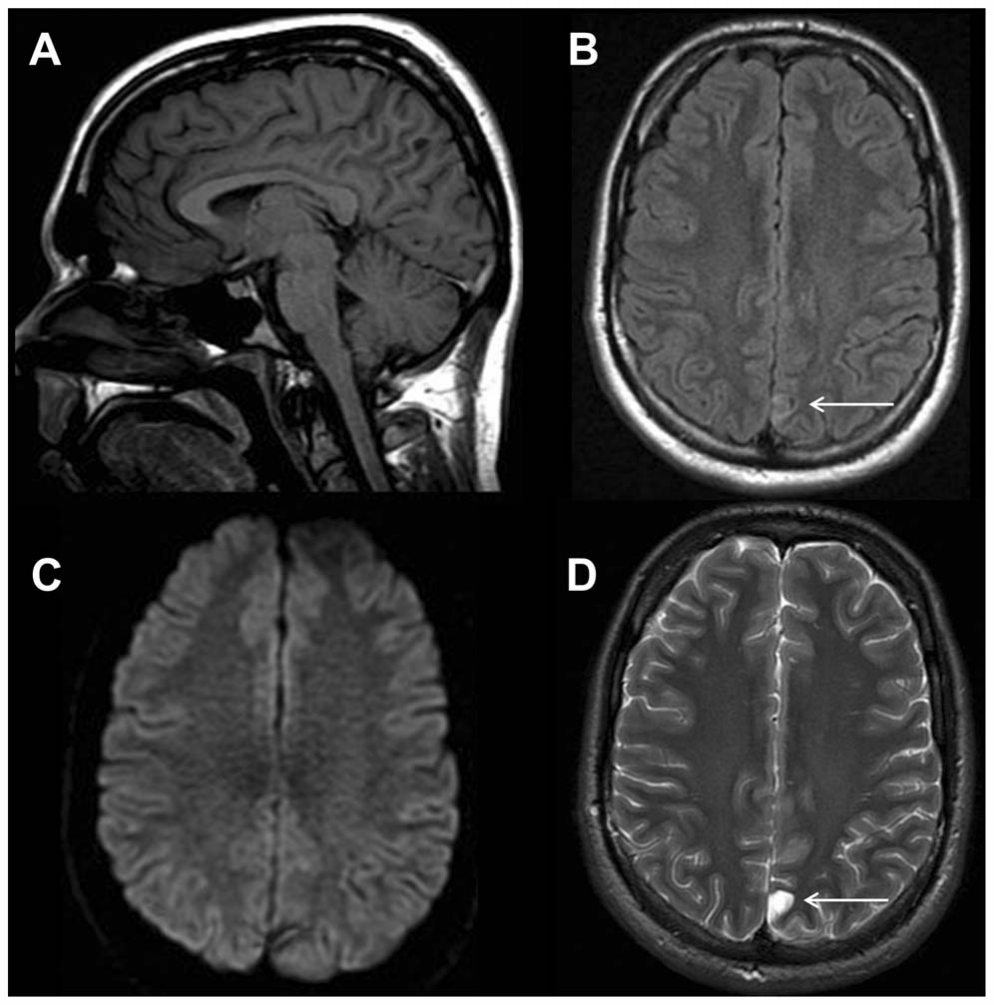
Cortical dysplasia versus dysembryoplastic neuroepithelial tumor in an 18 year old male with seizures. Sagittal T1-weighted image (A) shows no definite abnormality. Axial T2-weighted FLAIR image (B) demonstrates a subtle focal area of signal hyperintensity within the cortex and subcortical white matter of the left parafalcine parietal lobe(white arrow) with no corresponding restricted diffusion on the axial diffusion weighted trace (C) image. Axial T2-weighted image (D) again demonstrates the hyperintense lesion within the cortex and subcortical white matter of the left parafalcine parietal lobe (white arrow), which is compatible with cortical dysplasia or dysembryoplastic neuroepithelial tumor. This finding is subtle and was not seen prospectively when using the limited sequences. However, the lesion can be seen on the FLAIR images in retrospect. Furthermore, for seizure patients, additional sequences, including isotropic multiplanar sequences, could be added that would help in identifying subtle abnormalities.
